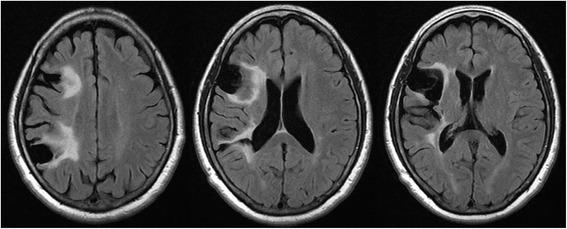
Brain MRI taken at the onset of camptocormia. Old infarcted lesions with tissue loss in the right pre-rolandic area, corona radiata, and postcentral gyrus are shown on FLAIR sequence
